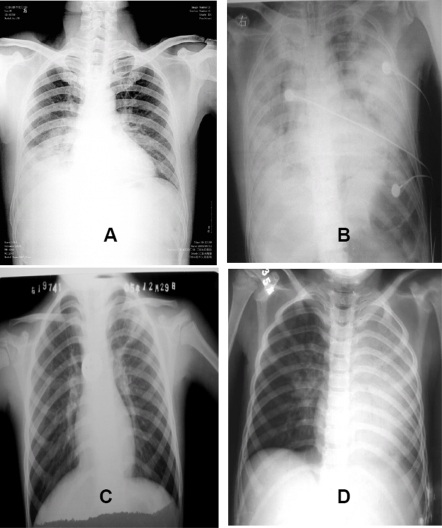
Progression of pulmonary disease in chest radiographs from adult (35-year-old male, Panels A day 6 and B day 23 of illness) and pediatric (6-year-old male, Panels C day 6 and D day 14 of illness) H5N1 cases.
